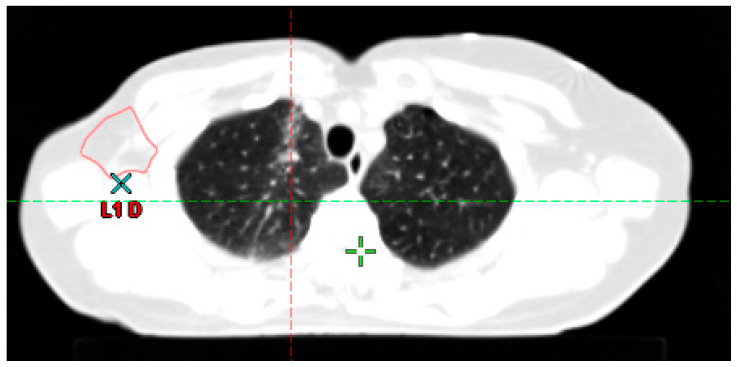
Radiation-Induced Fibrosis Post Right Breast Cancer Radiation Therapy. This figure presents a case of a patient who underwent radiation therapy for right breast cancer, with the subclavicular lymph node area included in the target volume. A lung CT scan, conducted six months post-treatment, shows an opacity in the right upper lobe indicative of radiation-induced fibrosis. It is crucial to distinguish this from potential lung infection.
